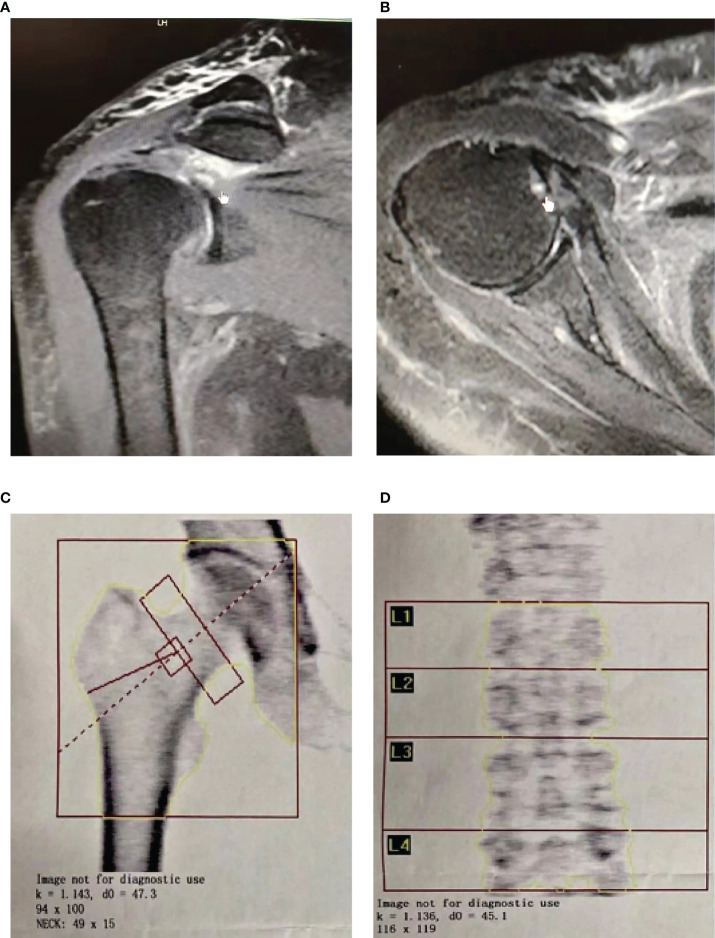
Rotator cuff tear with osteoporosis patients were defined by MRI (A, B) and DXA (C, D).
